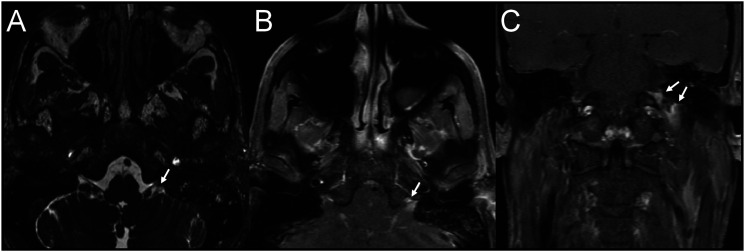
MRI with contrast and Fast Imaging Employing Steady- State Acquisition (FIESTA) sequencing. (A) Axial FIESTA sequence revealing obscuration of the jugular foramen (white arrow). Axial (B) and Coronal (C) T1 post-contrast images revealing enhancement of the left jugular foramen (white arrows).
